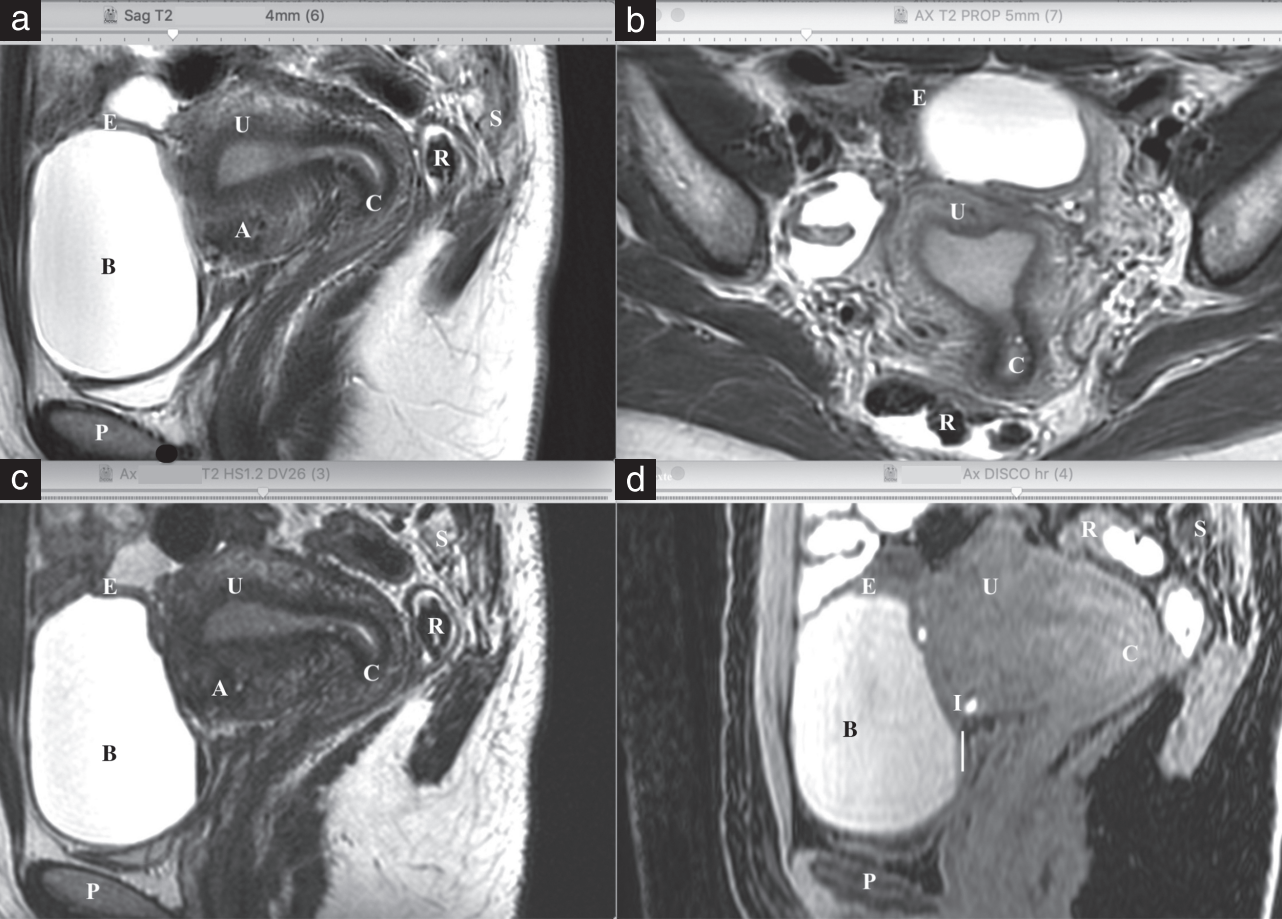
Sagittal two‐dimensional (2D) T2‐weighted (T2) fast‐spin‐echo (FSE) (a), axial 2DT2 FSE (b), reformatted sagittal three‐dimensional (3D) T2 (c) and 3D T1‐weighted with fat‐suppression (d) magnetic resonance imaging sequences in the same patient, displaying the different pelvic structures and organs: pubic symphysis (P), bladder (B), uterus (U), cervix (C), rectosigmoid colon (R) and sacrum (S). Note presence of endometrial cyst (E), superficial peritoneal implants (I) and external adenomyosis (uterine endometriosis) (A).
